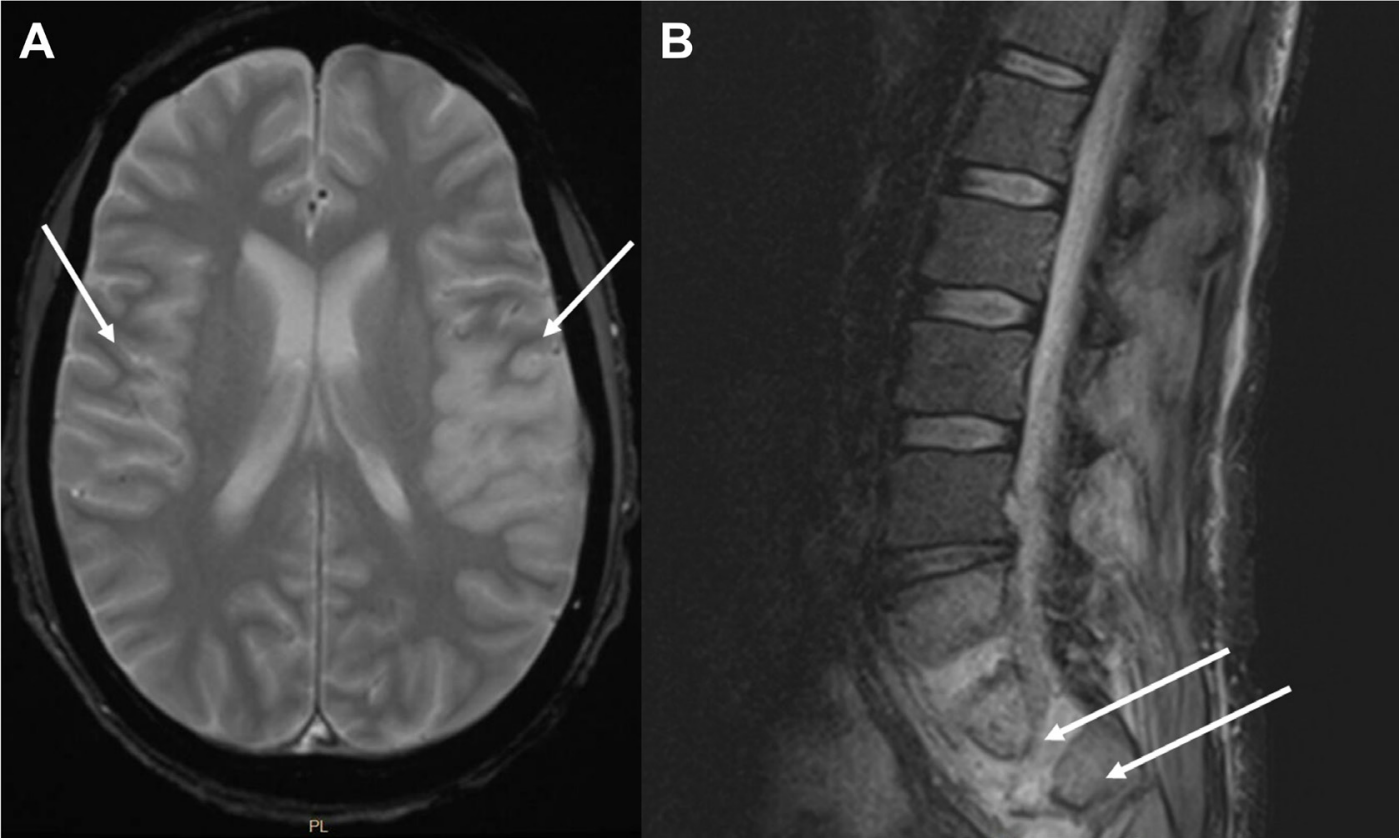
Brain and spinal cord infarctions and abscess. (A) Magnetic resonance (MRI) of brain with and without (w/wo) contrast with left and right middle cerebral artery infracts and (B) L spine MRI showing L5-S1 discitis-osteomyelitis with intramuscular paraspinal abscess at the L5 and S1 levels.
